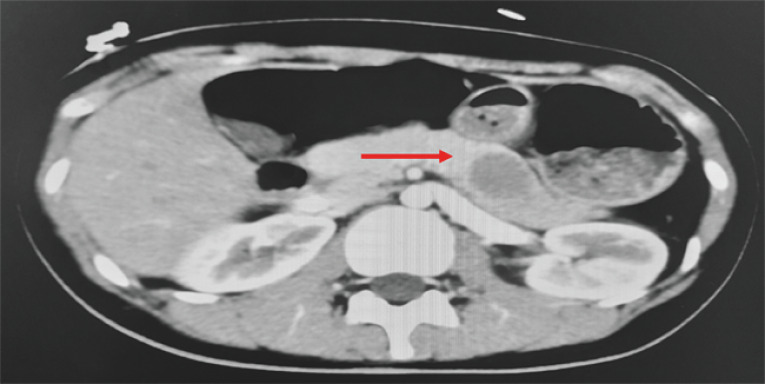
Contrast-enhanced computed tomography scan revealed a round, well-defined, hypodense lesion (red arrow) within the pancreatic body.
